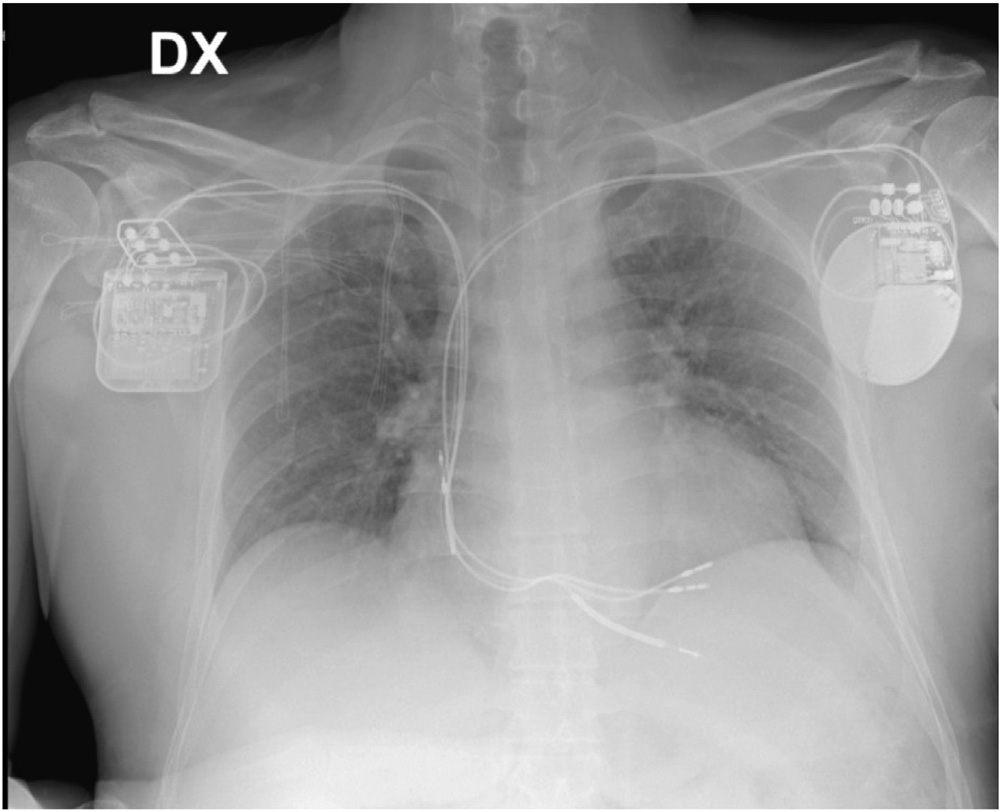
Chest radiograph of the patient. The figure shows cardiac contractility modulation (CCM) device on the right side and implantable cardioverter-defibrillator (ICD) DR on the left side. DX indicates right site; DR indicates a dual chamber device. Adx lead = right atrial lead for ICD; CCM1-RV = lead reference ventricular CCM; CCM2-LS = lead local sense CCM; ICD lead = right ventricular lead for ICD.
